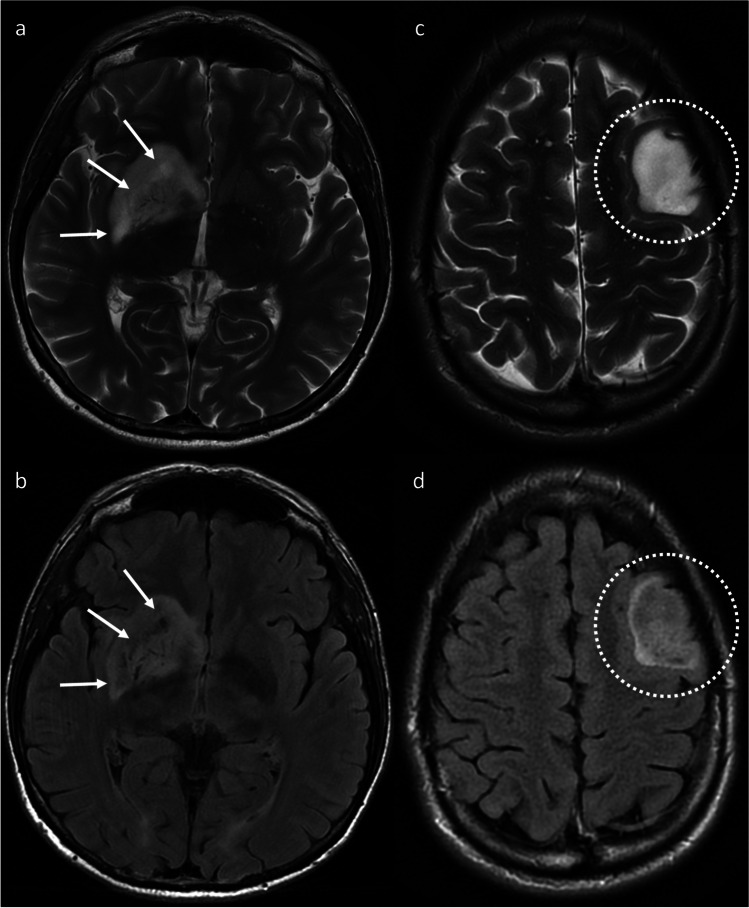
Axial T2WI (top row) and FLAIR (bottom row) images of two patients with IDH^mut^/1p19q^int^ LGG. The first patient (a, b) has a tumor centered on the right lentiform nucleus, demonstrating 25–50% T2-FLAIR mismatch. The arrows highlight areas with high T2 signal and substantially lower signal on FLAIR. The second patient (c, d) has a left frontal lobe tumor with > 50% T2-FLAIR mismatch and well-defined tumor margins
